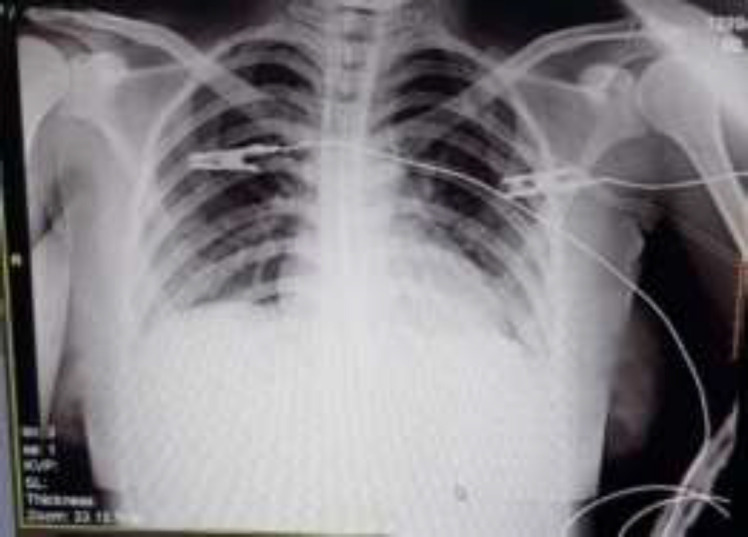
Chest x-ray no findings suggestive of COVID-19 pneumonia
